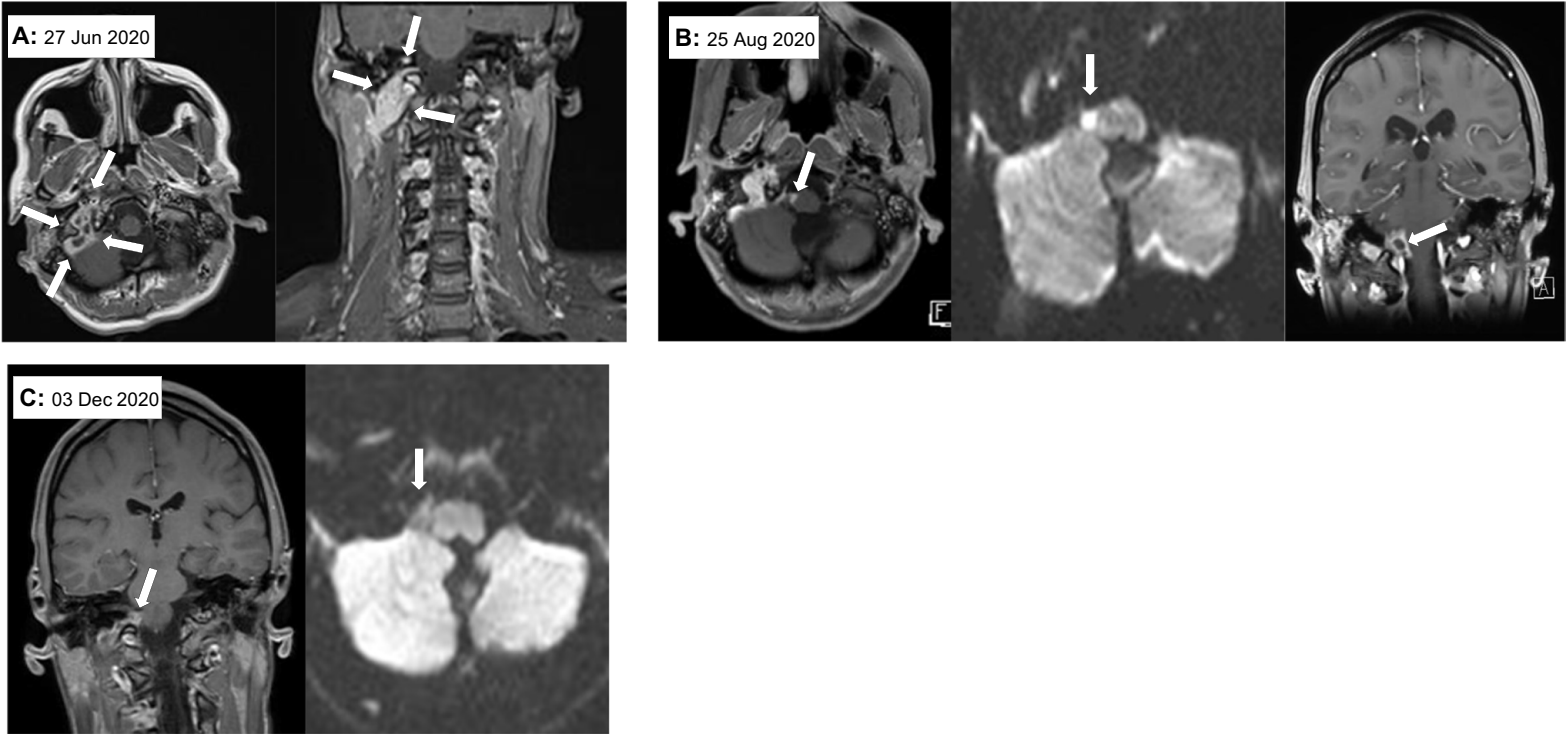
MRI findings: White arrows point A: toward the septic thrombosis of the right sigmoid sinus/internal jugular vein at axial T1_tse (left) and coronal T1_tse_dixon with fat saturation (right) post contrast enhancement, B: toward an abscess formation adjacent to the medulla oblongata showing circular contrast enhancement at axial T1-3D_MPRAGE (left) and coronal reconstruction of T1_3D_MPRAGE (right) as well as and high central signal on DWI imaging (middle), C: toward post-inflammatory scar tissue located in the region of the previous abscess formation that shows no circular contrast enhancement at coronal reconstruction of T1_3D_SPACE (left) and no high central signal on DWI (right) anymore
